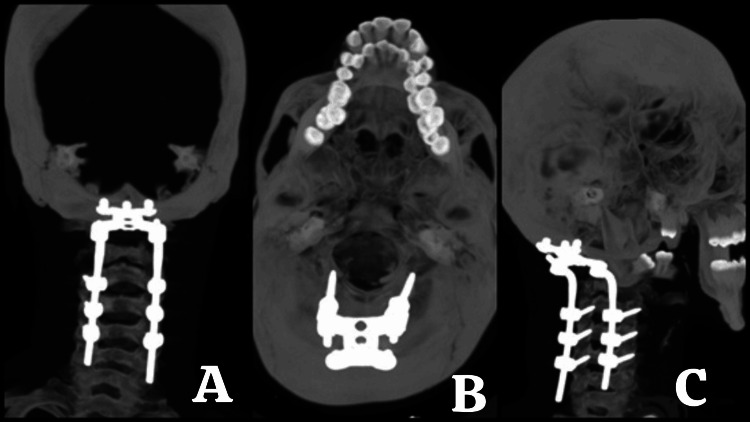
3D reconstruction from skull CT with bone window A. Coronal view showing midline occipital screws and bilateral lateral mass or pedicle screws from C3 to C5 with rods extending caudally. B. Axial view at the craniovertebral junction demonstrating symmetric placement of the occipital plate and screws, confirming proper midline fixation. C. Sagittal view displaying the longitudinal alignment of the occipitocervical construct from the occiput to C6 with maintained anatomical alignment and hardware integrity.
